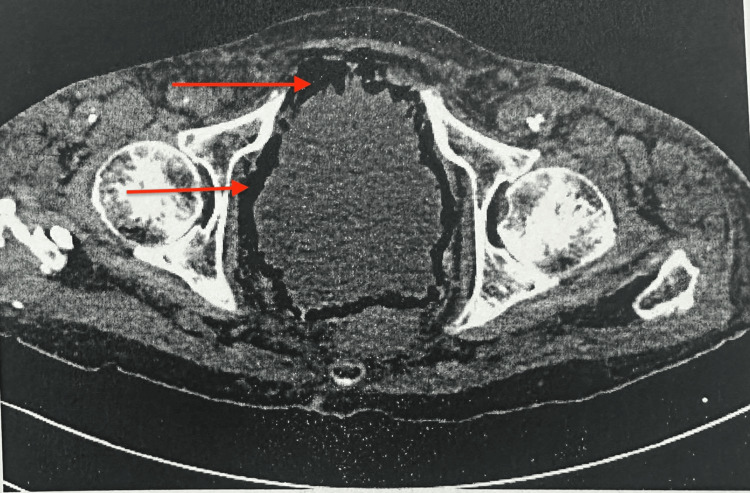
Axial CT at the pelvic level. The bladder is markedly distended, with intramural gas along the anterior and lateral wall, suggestive of emphysematous cystitis. Arrows indicate intramural gas. CT: computed tomography
